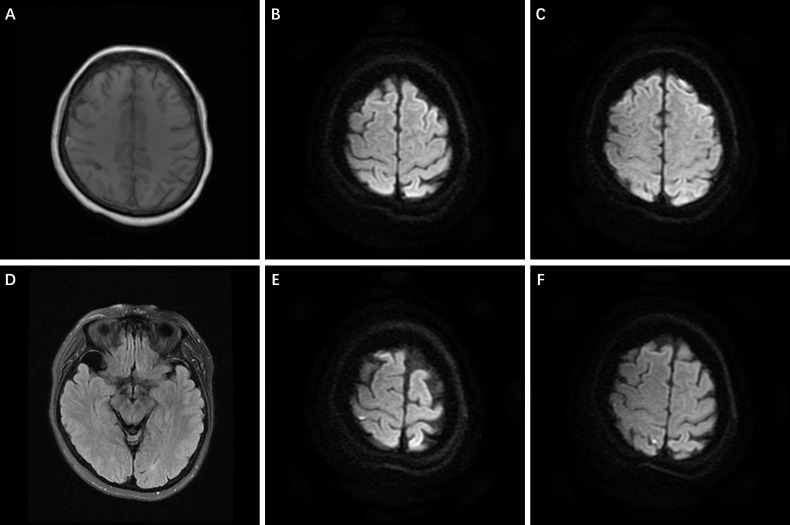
Head MRI+DWI on the fourth (A-D) and sixth (E, F) days of hospital admission. (A) Suspected minor SAH in the right frontal-parietal lobe, (D) right temporal pole arachnoid cyst, (E, F) new infarction in the cortical area of the right parietal lobe, and (B, C) comparison with the images from two days prior.
